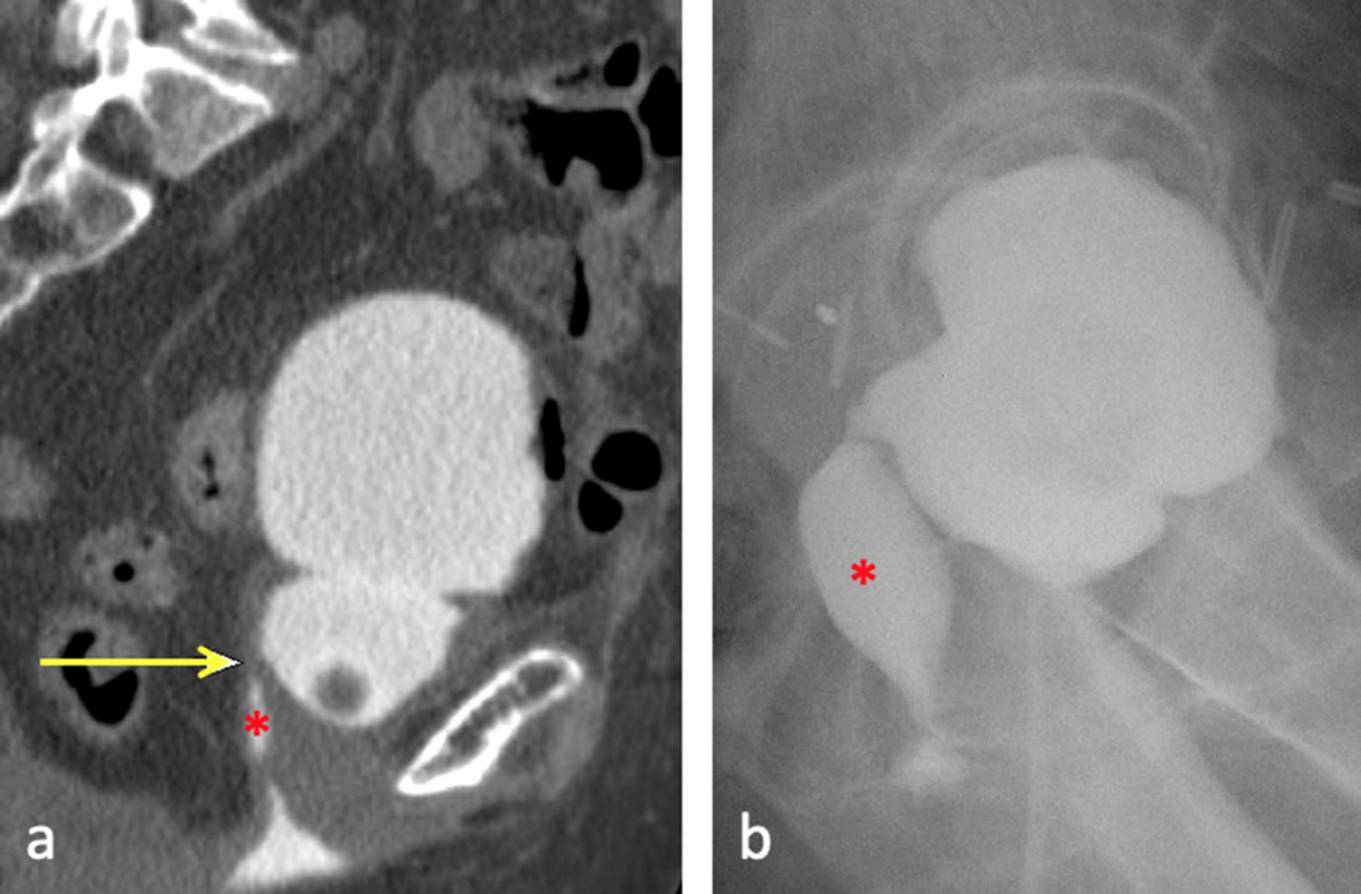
71-year-old female with cervical cancer status post hysterectomy and pelvic radiation. Sagittal CT Cystogram a demonstrates contrast in the vagina (*) with a fistulous connection to the bladder (arrow), representing a vesicovaginal fistula. Lateral cystogram c demonstrates injection of contrast into the bladder and immediate leakage of contrast through the vagina (*) in the region of the vaginal cuff, compatible with vesicovaginal fistula
